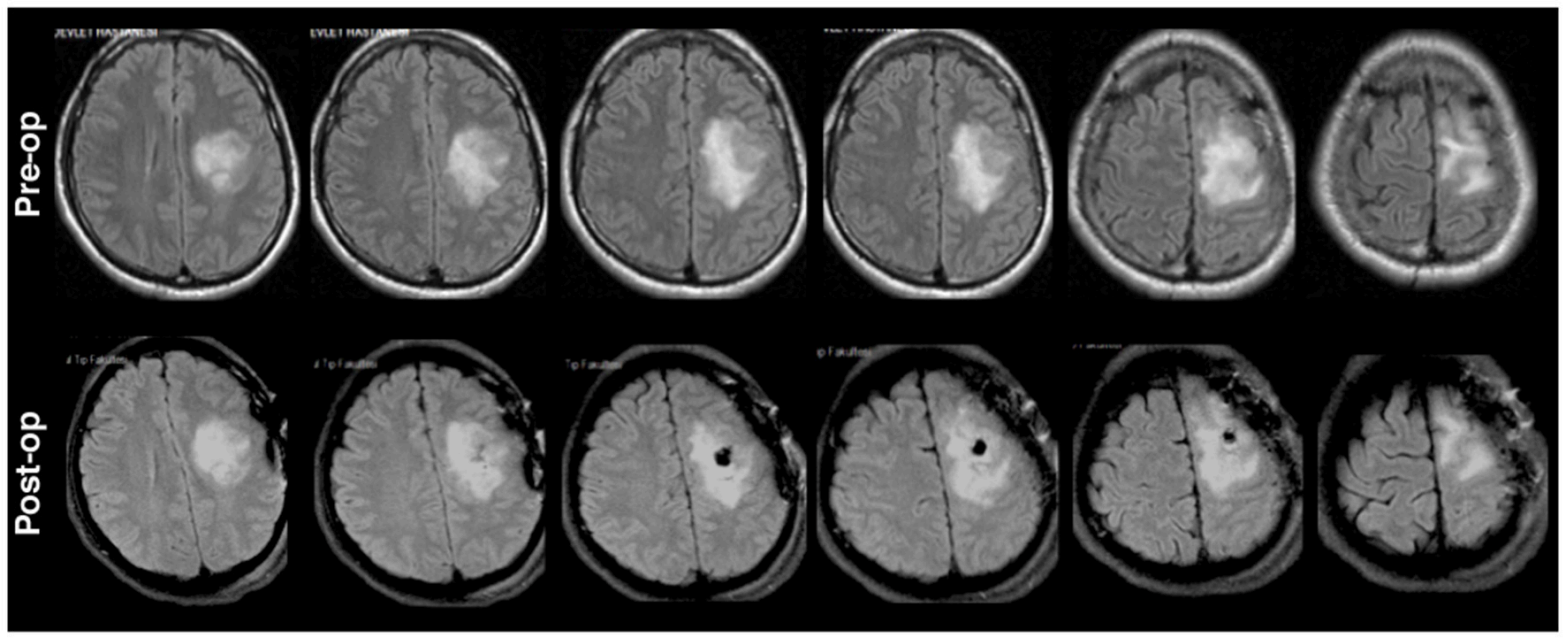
Preoperative (upper) and postoperative (lower) axial MRI FLAIR sequences for P2. Surgery was terminated with incomplete resection due to determination of right hand weakness.
